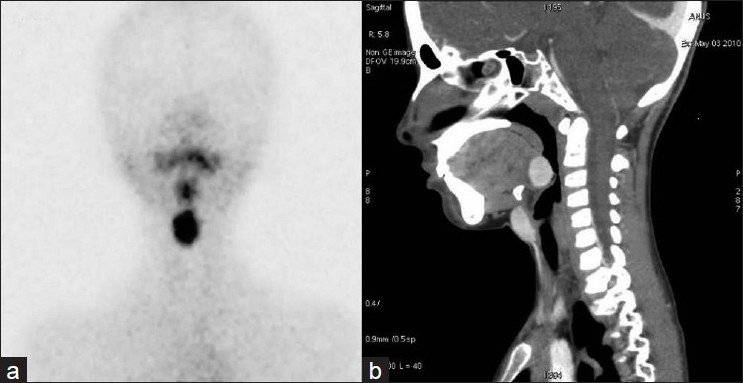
(a, b) Thyroid scan and CT image (sagittal section) showing dual ectopia - sublingual and subhyoid - with absence of normal thyroid
